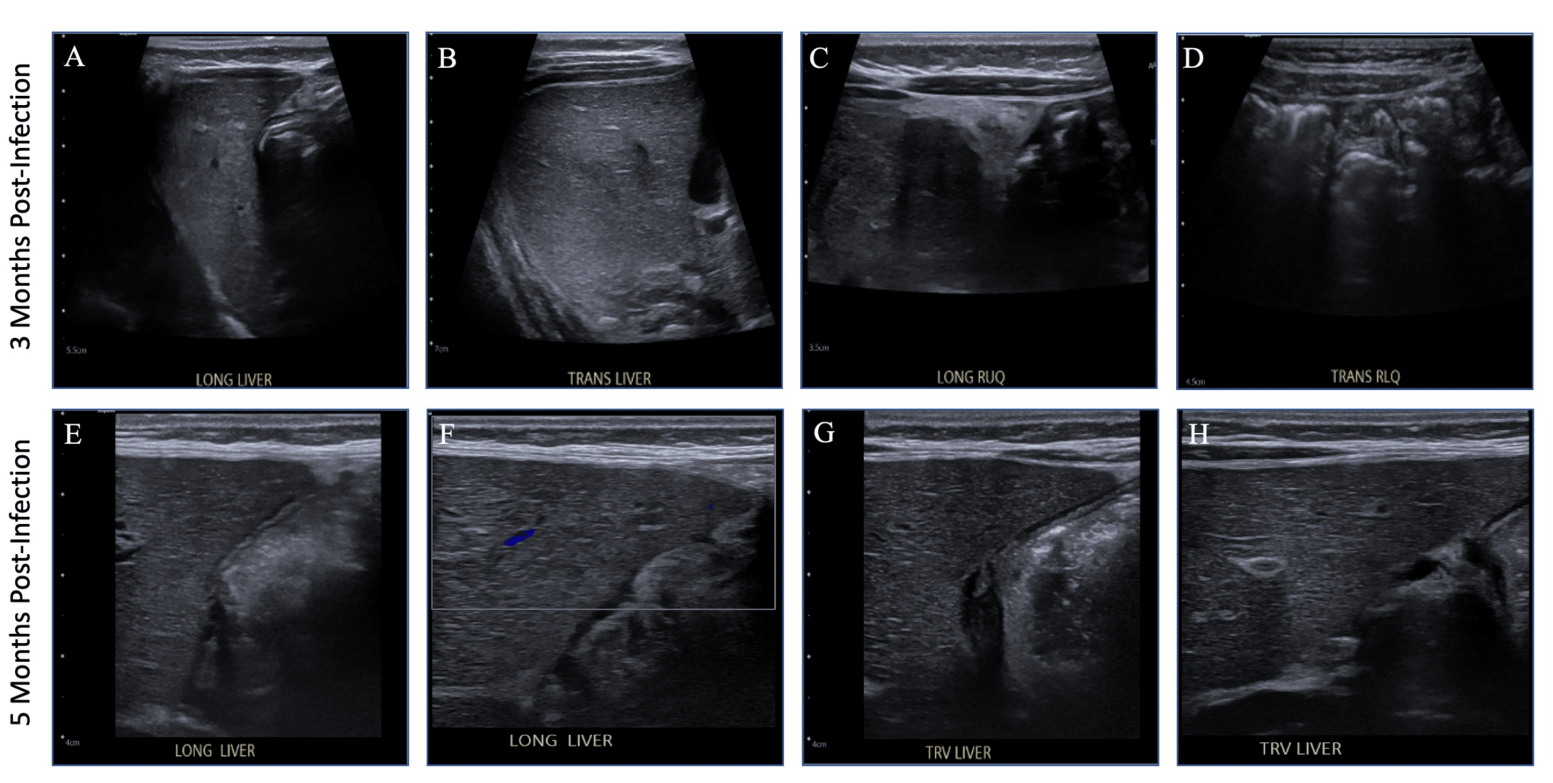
Repeat ultrasound three and five months post-infection. At three months post-infection, (A) liver long axis;  (B) liver transverse axis; (C) right upper quadrant long axis; (D) right lower quadrant transverse axis, views demonstrating near complete resolution of hepatic abscesses. At five months post-infection (E, F) liver long axis; (G, H) and liver transverse axis views demonstrating complete resolution of hepatic abscesses.
